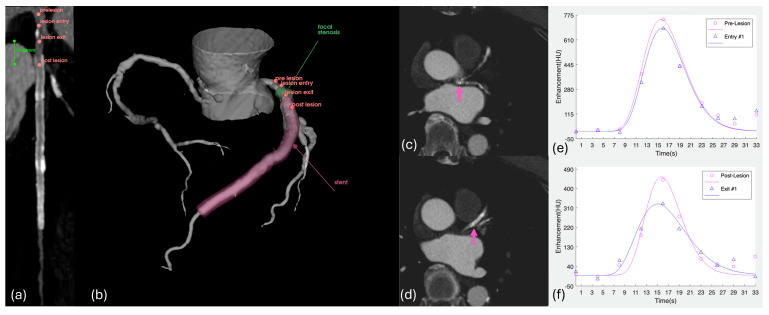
A 74-year-old female (patient 11 in Table 1) whose left anterior descending artery (LAD) had a 25% focal stenosis in the proximal segment followed by a stent that extended to the distal segment. The FFR value measured in the mid-LAD segment (coronary segment #7) had a CT-DAI of 0.85, which was close to the value of 0.86 acquired with the invasive catheter-based method. (a) Straightened lumen view of the LAD with the sampling regions of interest (ROIs) at the pre-lesion, lesion entry, lesion exit and post-lesion sites. The post-lesion ROI was placed within the stent in the mid-LAD segment to match the invasive FFR measurement site. (b) Sampling ROIs shown in the three-dimensional image of the LAD. (c) Pre-lesion ROI (pink arrow) and (d) post-lesion ROI (pink arrow) shown in the axial CT myocardial perfusion image at one time point. (e,f) The plots show the raw data points sampled from the ROIs shown in (a–d) and the corresponding fitted coronary time-enhancement curves.
